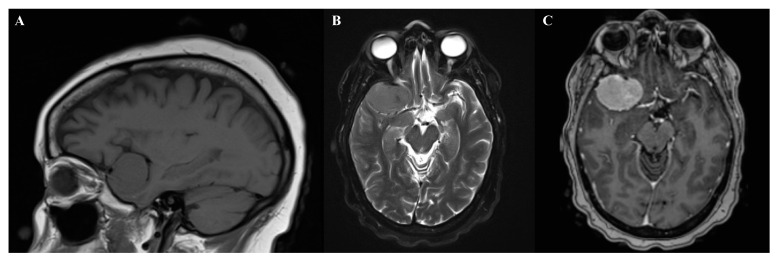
Magnetic resonance imaging of right sphenoid wing meningioma. (A) Sagittal T1. (B) Axial T2. (C) T1+gadolinium contrast.
